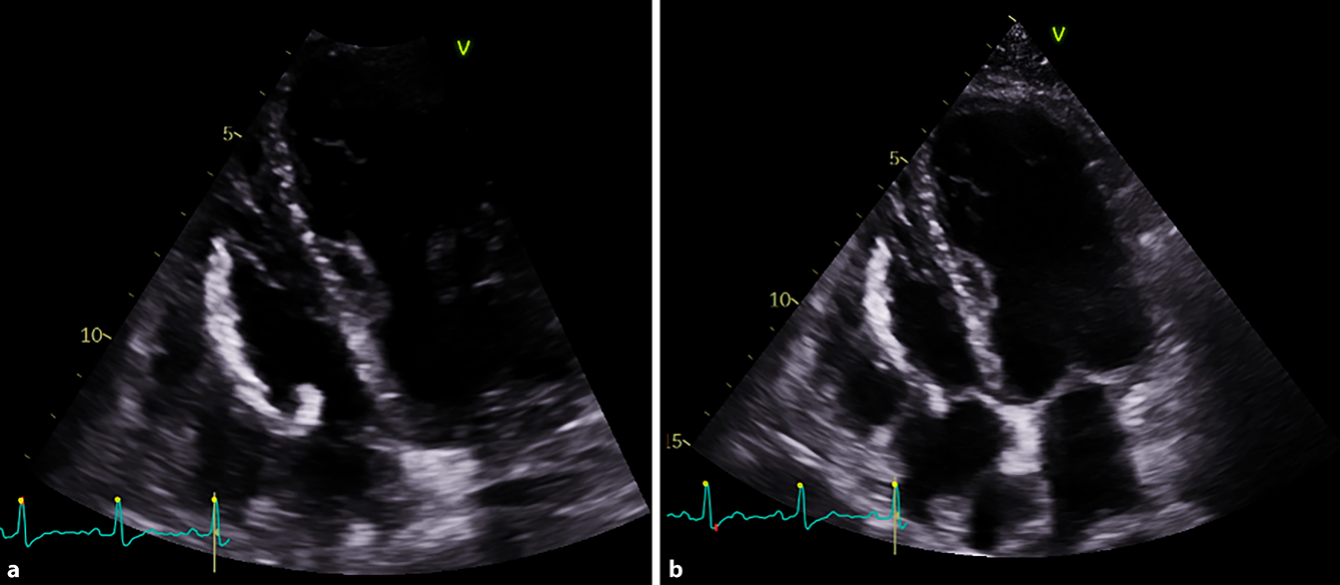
Transthoracic echocardiography. a Four-chamber right-ventricle focused view showing hyperechogenic, filiform, anomalous mass (8 × 5 mm), with mobile tip curving inside tricuspid valve. b Four-chamber view showing attachments of mass to right ventricular wall and below tricuspid valve
